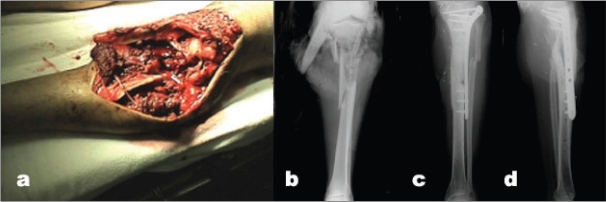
(a) Clinical photograph shows IIIC tibial plateau and shaft fracture. (b) Anteroposterior X-ray of the leg shows type IIIC tibial plateau and shaft fracture. (c,d) Anteroposterior and lateral radiograph at 6-year follow-up shows fracture union.
